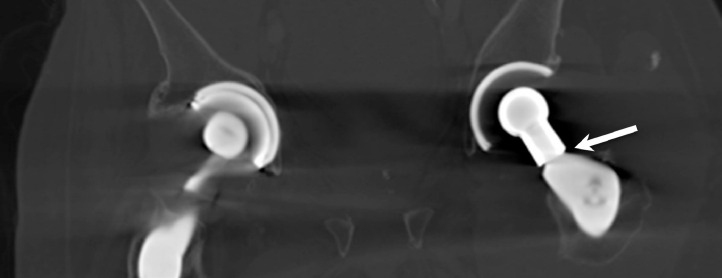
Coronal CT image of the pelvis with bone windows demonstrates modular design of the left total hip arthroplasty, with the femoral head-neck interface or trunnion indicated by the arrow.
